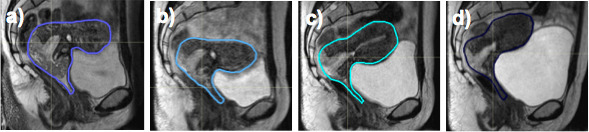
Changes in clinical target volume position during cervix radiotherapy as seen on MRI at (a) week 0, (b) week 2, (c) week 3 and (d) week 4.
